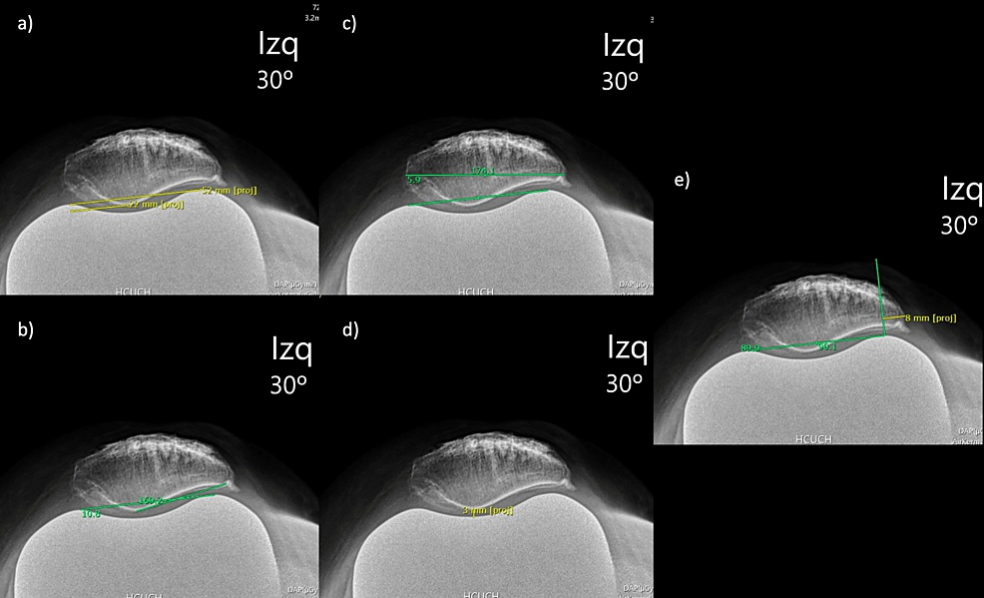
Axial knee radiograph (a) Patellar lateralization distance; (b) Opening lateral patellar angle; (c) Patellar tilt angle; (d) Patellofemoral joint space; (e) Lateral patellar overhanging
